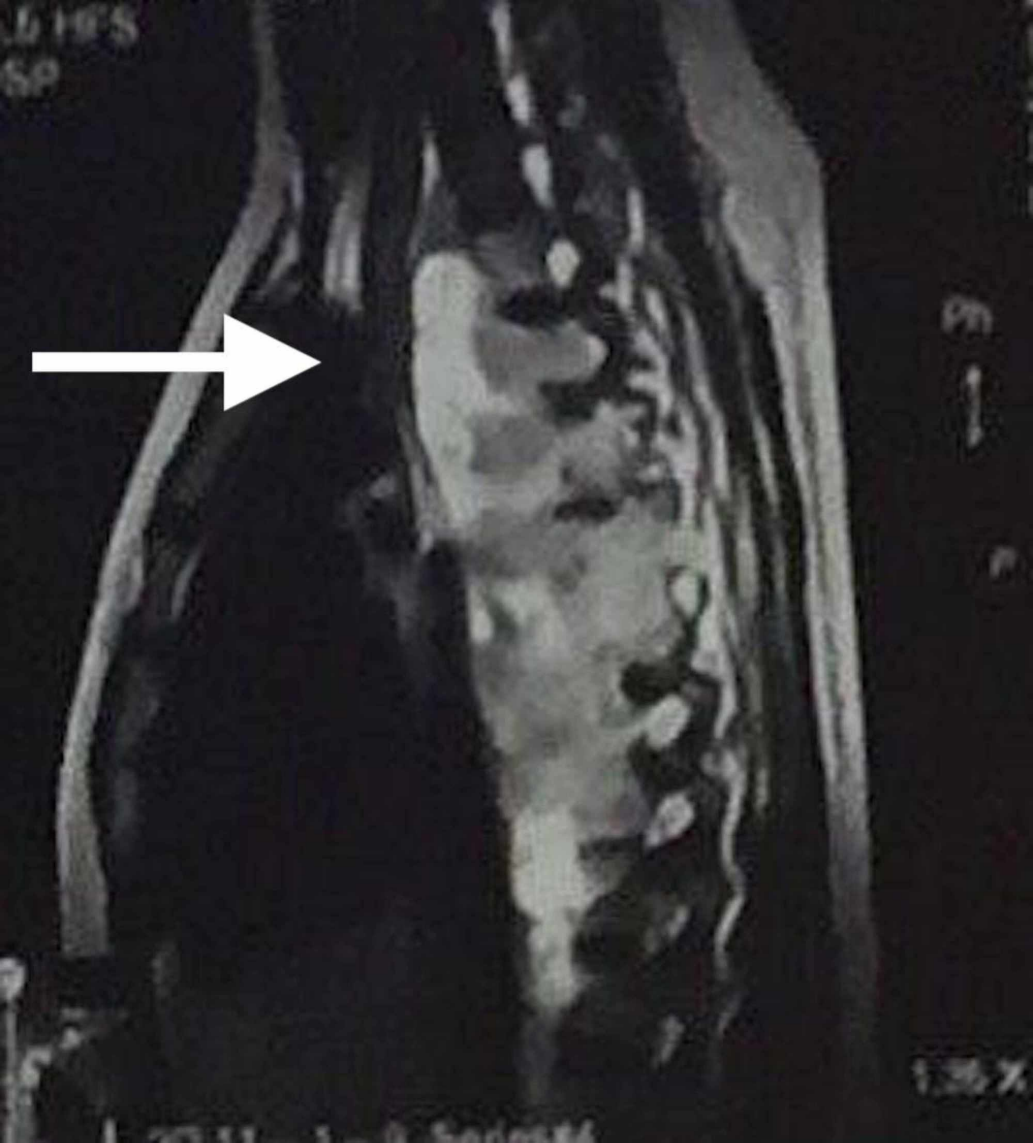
MRI sagittal view Showing paravertebral lesion, infiltration of the vertebral bodies.
